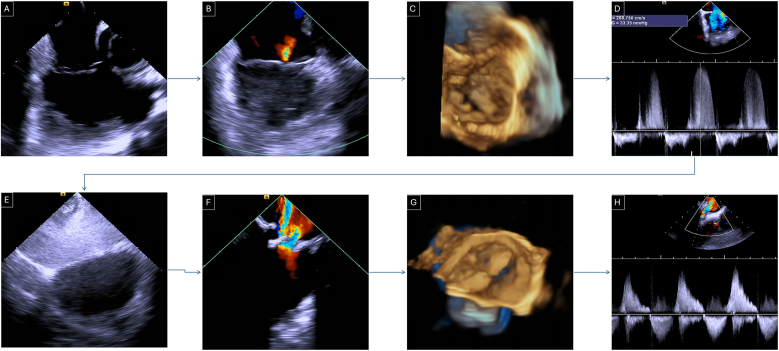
ICE protocol: A- “home view” looking at the tricuspid valve, RV function and pericardial effusion in 2D. B- Color Doppler on the tricuspid valve to check for TR. C- 3D image of the tricuspid valve. D- CW Doppler to check for tricuspid valve peak instantaneous gradient. E− Looking at the interatrial septum with agitated saline test showing agitated saline in the RA and clear LA. F- Looking at the pulmonary valve with color Doppler showing severe regurgitation. G- 3D of the pulmonary valve. H – CW across the RVOT. CW, continuous wave Doppler; ICE, intracardiac echocardiography; LA, left atrium; RA, right atrium; RV, right ventricle; RVOT, right ventricular outflow tract.
